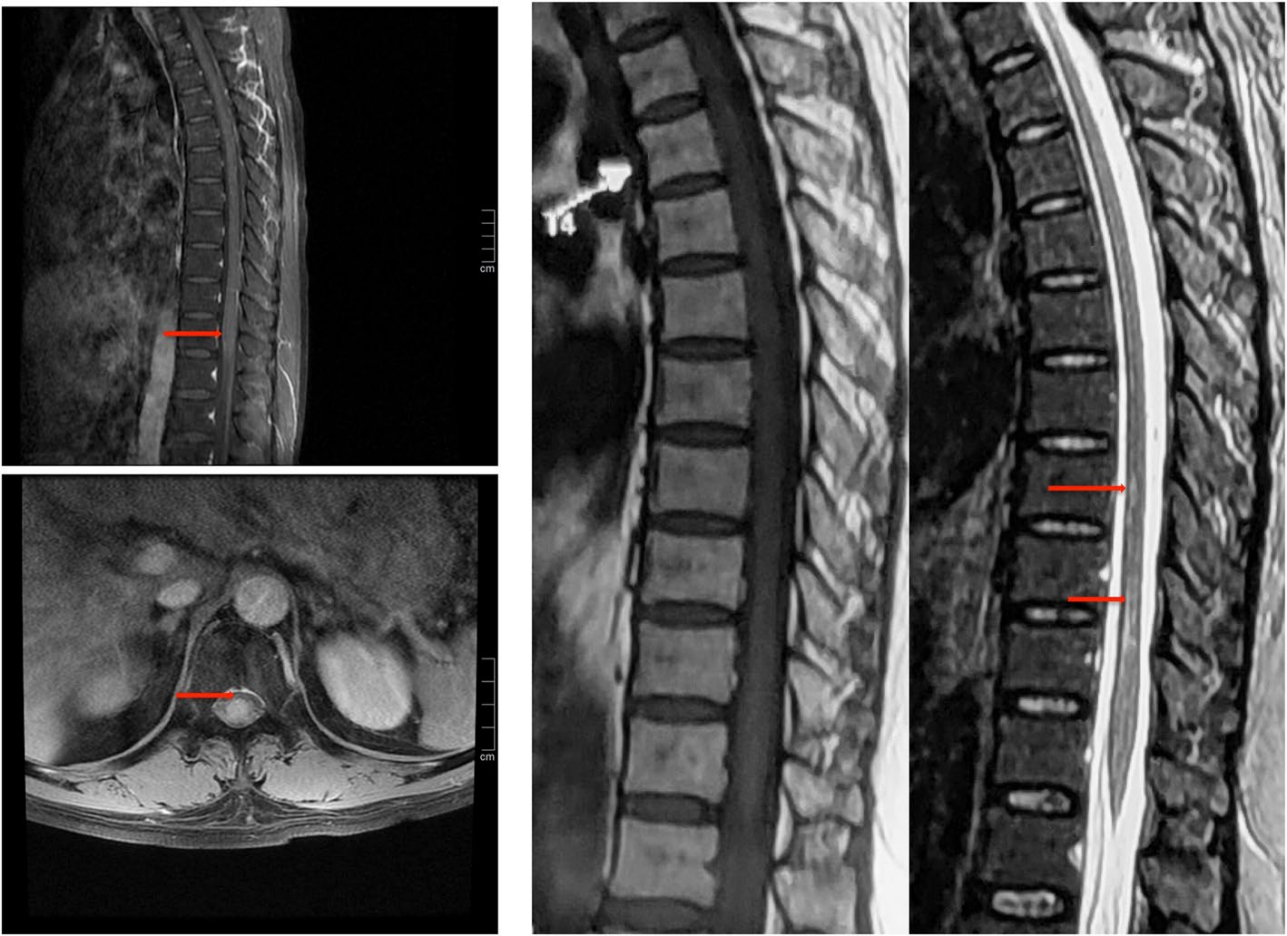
Spinal MRI revealed a hyperintense T2 lesion in the spinal cord extending from T8 to T11, and enhancement MRI indicated small patchy and strips hyperintensities in the spinal cord and meninges. The red arrow refer to the injuries
